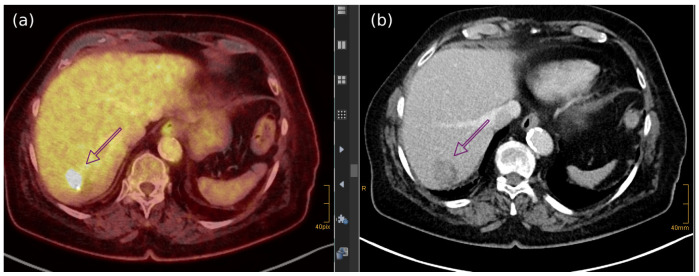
Hepatic metastasis from Merkel cell carcinoma. (a) PET/CT image shows an FDG–avid lesion in segment VII of the liver (purple arrow). (b) Corresponding CT image reveals approximately 3 cm enhancing nodule with irregular margins, no signs of central necrosis or capsular retraction.
